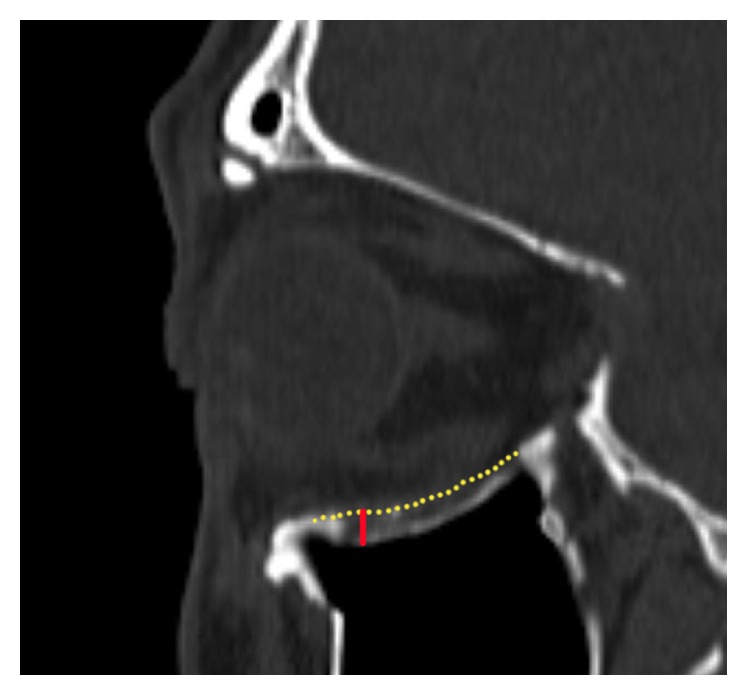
Sagittal view of the CT from the 14-month follow-up of a patient reconstructed with resorbable plate only is shown to depict how the postoperative results were evaluated. The dotted yellow line depicts the normal baseline orbital floor inferred from the contralateral noninjured orbit, and the red line depicts the greatest height discrepancy between the imaginary normal yellow dotted line and the actual reconstructed orbital floor. The area between the yellow line and actual orbital floor was integrated to calculate the increase in orbital volume after surgery.
